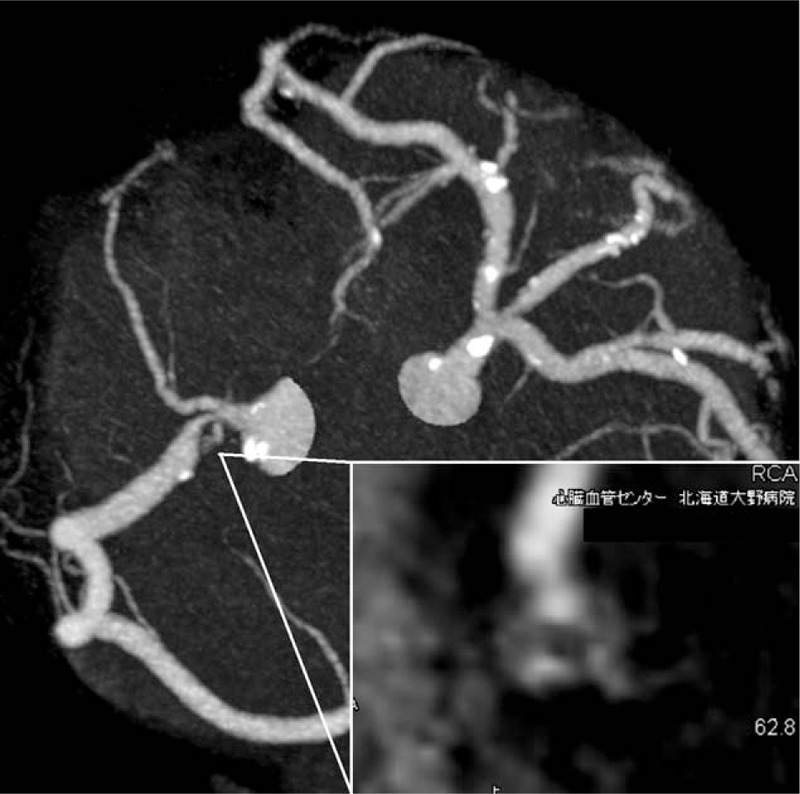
Coronary computed tomography angiography at 1-year follow-up showing an eccentric tight stenosis with a “Napkin-ring sign” at proximal RCA. RCA = right coronary artery.
